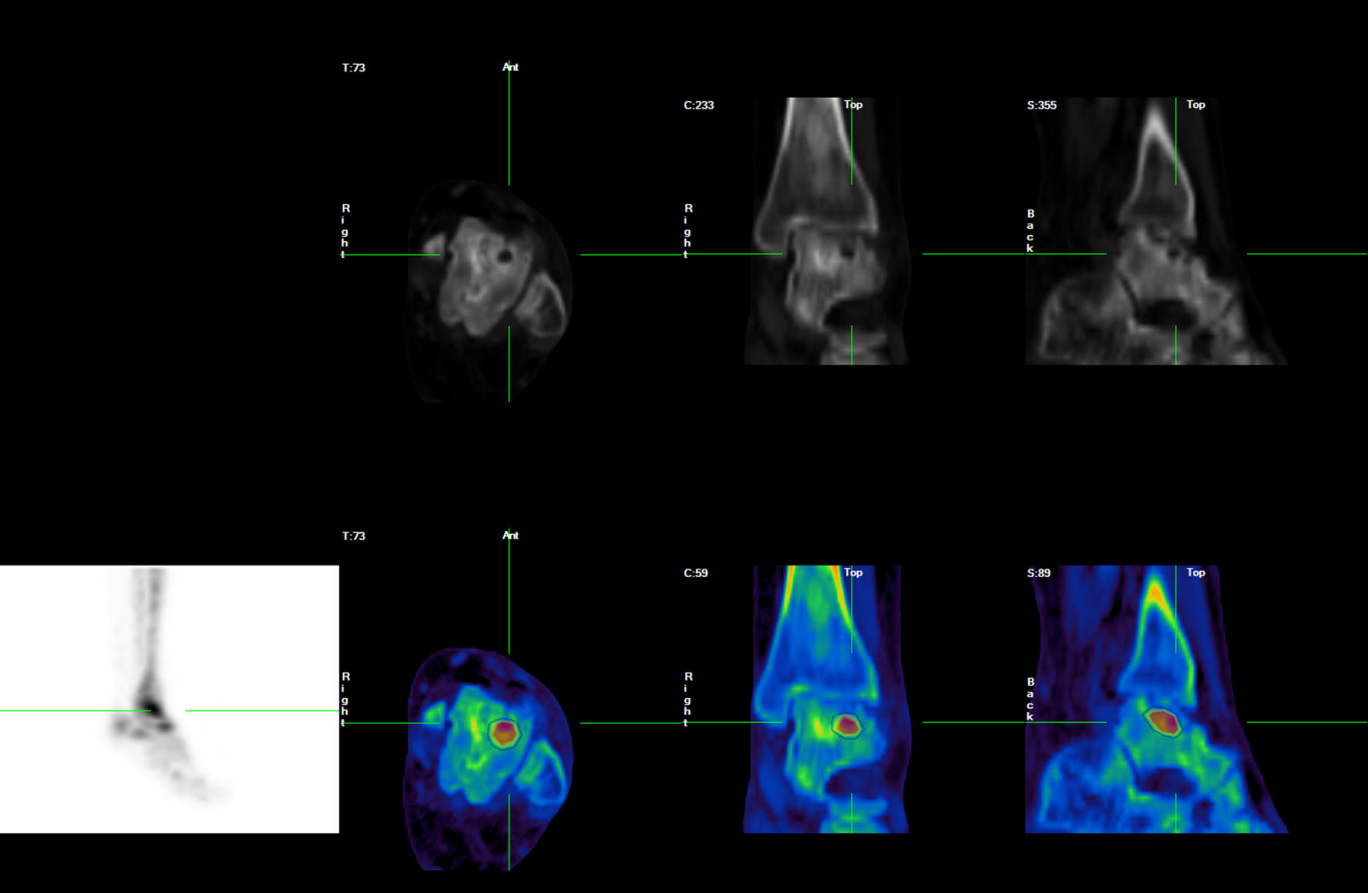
SPECT-CT images show a well-defined lesion with a central radiolucent area surrounded by a dense sclerotic rim and sinus tract formation together with a fracture line in the proximal left talus. These findings are consistent with a diagnosis of Brodie’s abscess in the proximal left talus
